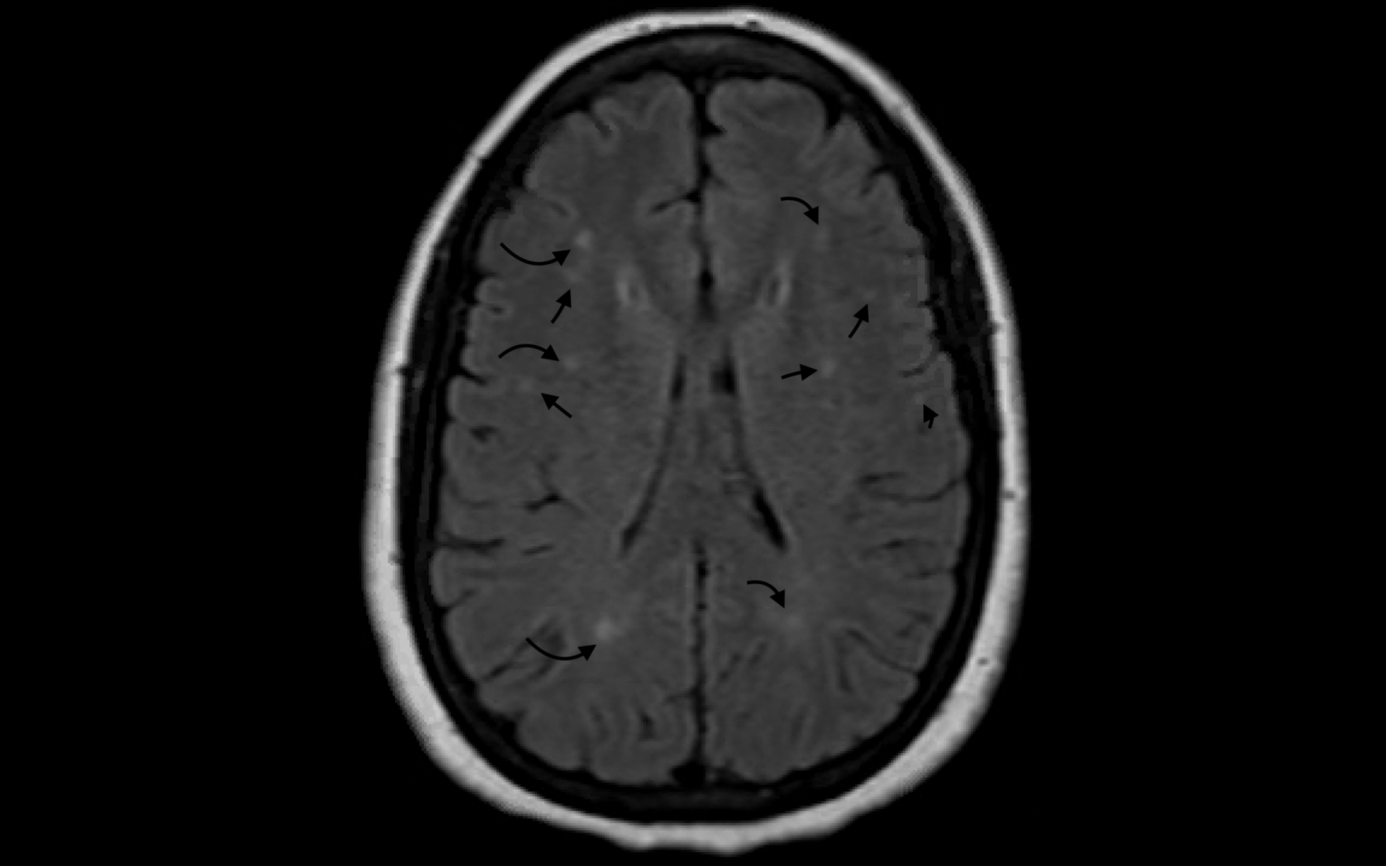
MRI of the brain Stable extensive white matter changes without the presence of acute infarction MRI: magnetic resonance imaging
